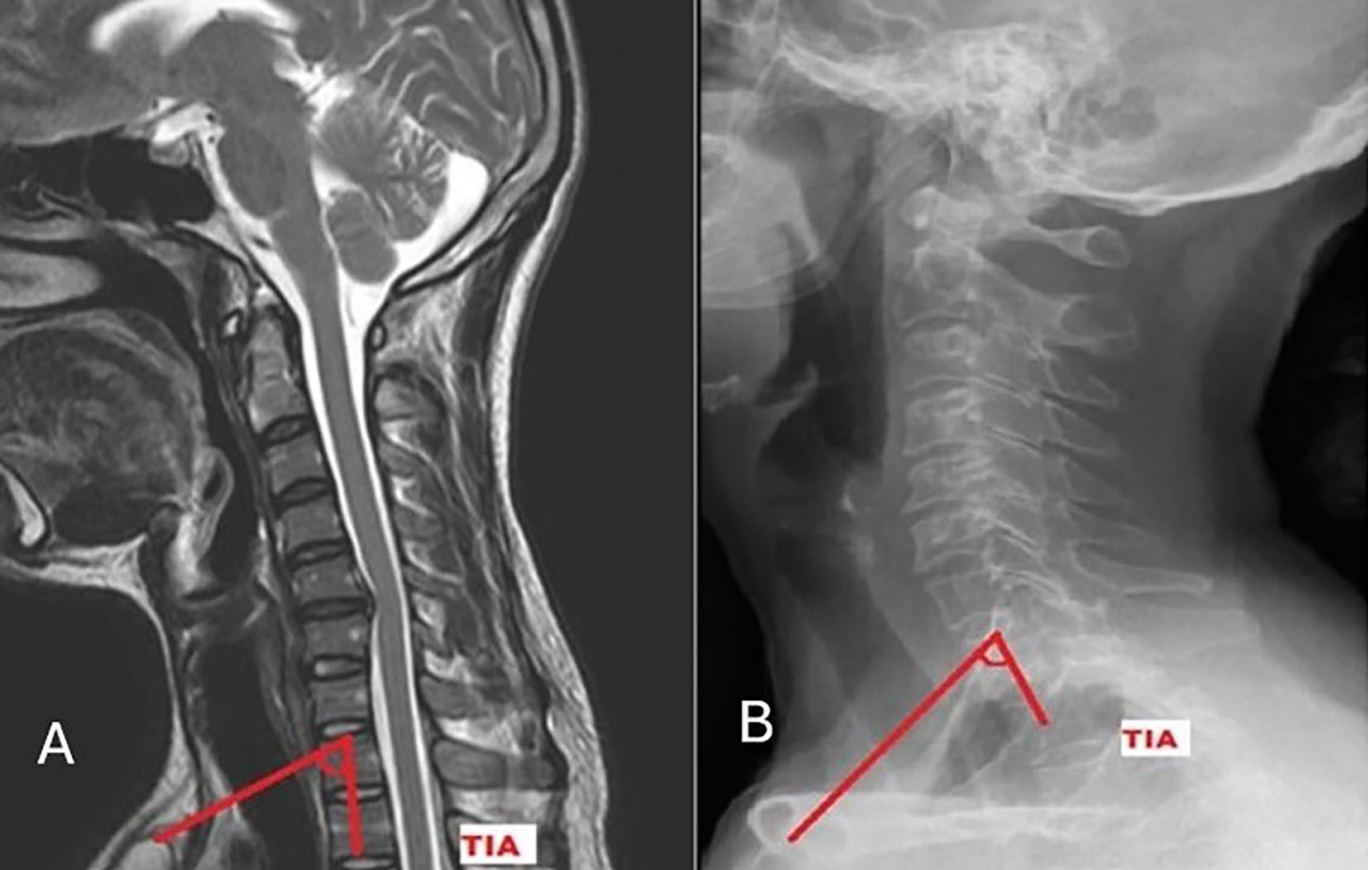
Measurement of Thoracic inlet angle on MRI (A) and radiograph (B).
